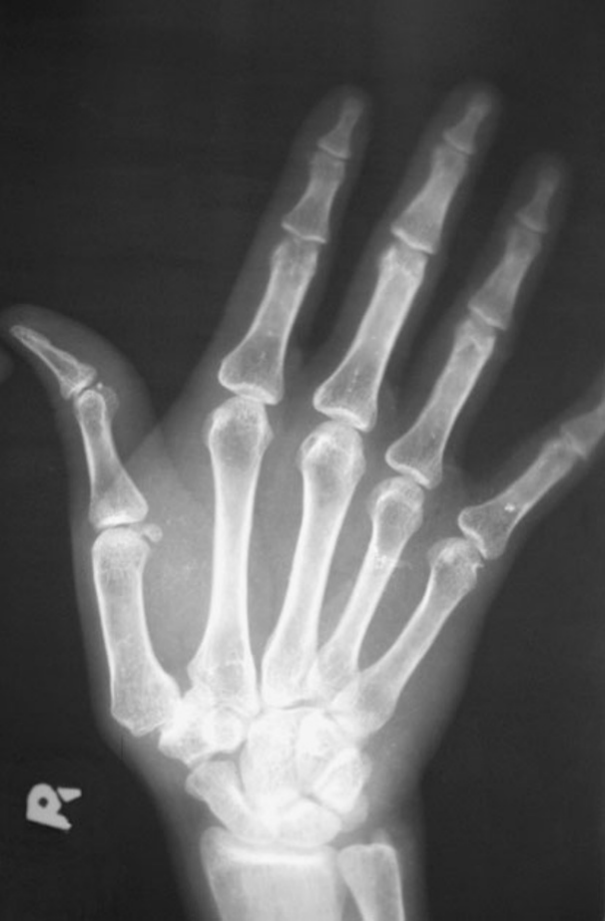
Preoperative radiograph showing subluxation of carpometacarpal joint of the right thumb
